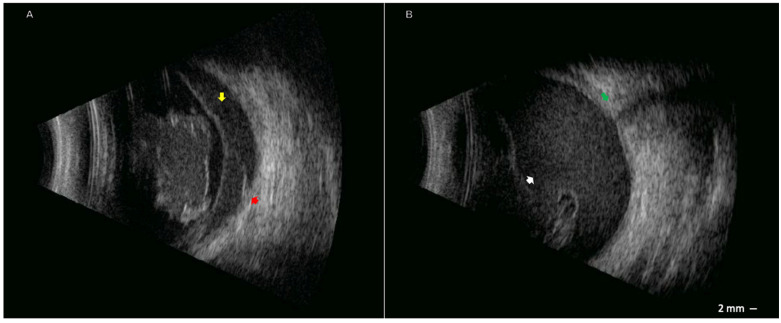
Ocular ultrasound was performed eight months after symptom onset. (A) Choroidal thickening (red arrow) and subhyaloid opacities (yellow arrow). (B) Dense vitreous opacities (white arrow) with a normal-appearing optic nerve (green arrow).
